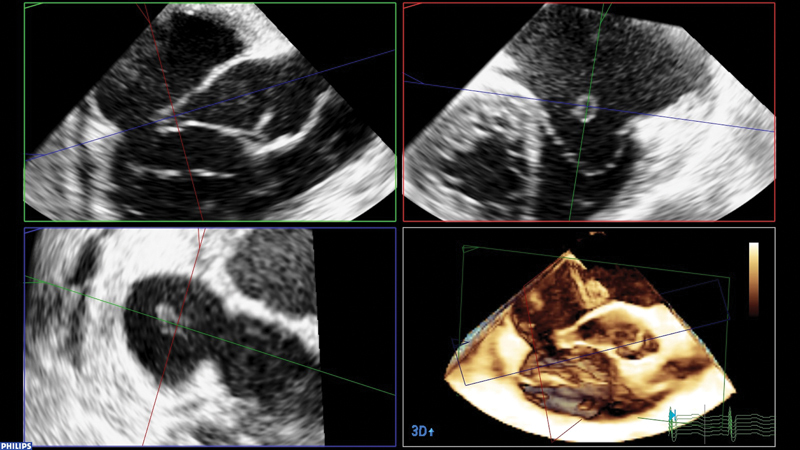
Transesophageal echocardiography delineating the anatomy in different planes and three-dimensional view. Note the elongated sinus of Valsalva and the opening toward the right atrium.
